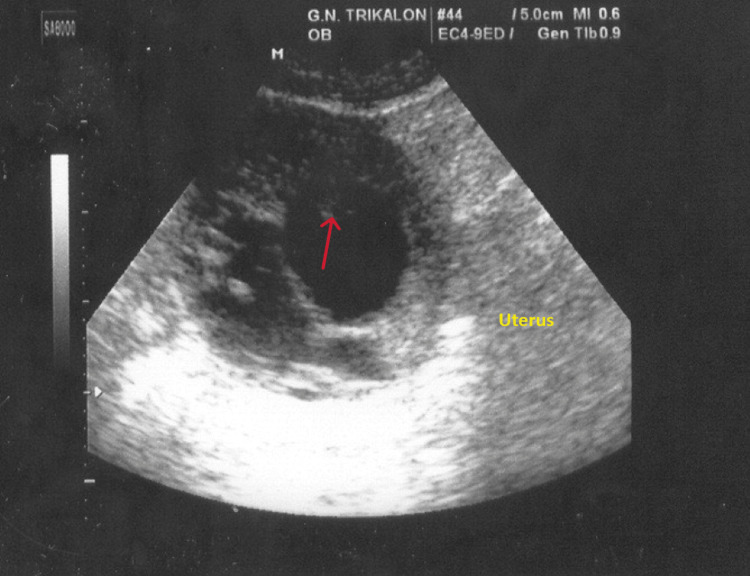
Transvaginal ultrasound imaging showing an interstitial ectopic pregnancy The body of the uterus is visible, as is the presence of a gestational sac with fetal elements without cardiac function (red arrow) in contact with the body of the uterus.
